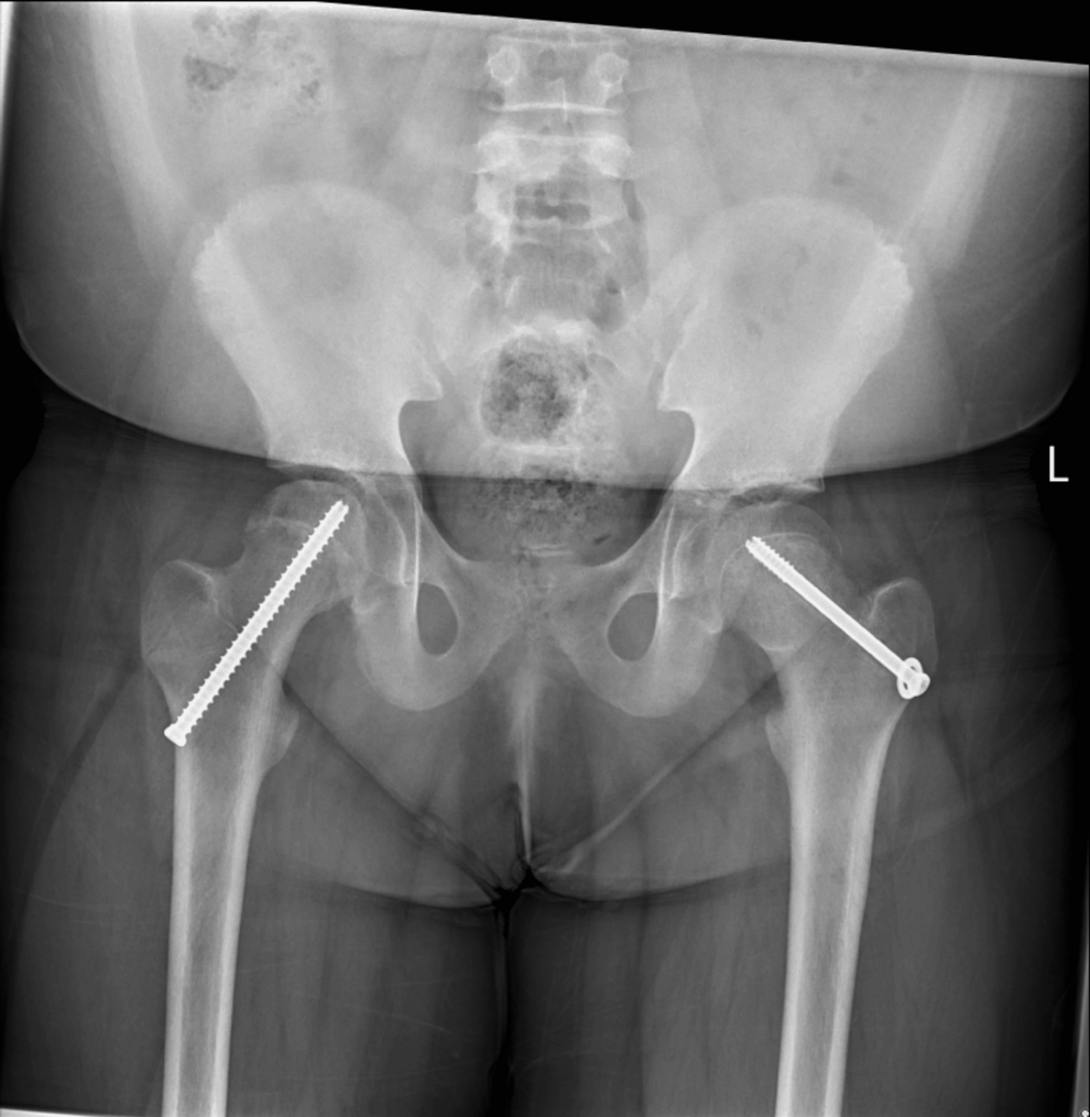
Post-operative X-ray pelvis (AP view) showing pin fixation in bilateral hips. AP: Anteroposterior
